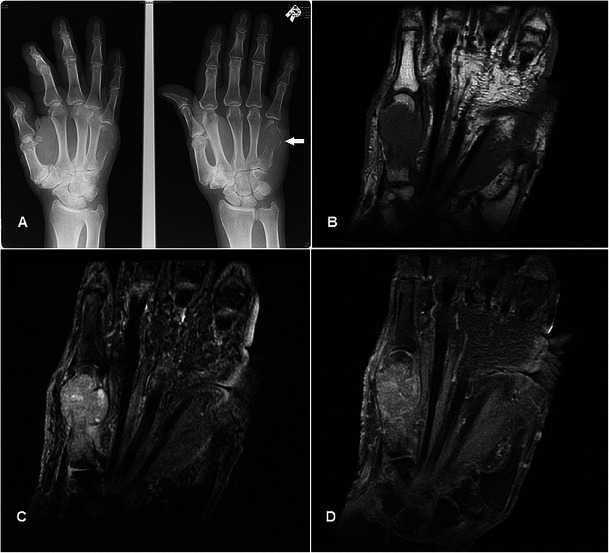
Bony metastasis in a 67-year-old male with a history of adenocarcinoma of the lung, presenting with a 2-week history of swelling and pain over the medial aspect of the right hand dorsum. (a) Plain radiograph shows permeative destruction of the fifth metacarpal (arrow). (b) T1w sequence shows destruction of the fifth metacarpal with infiltrative soft tissue, predominantly of heterogeneous intermediate signal. (c) The lesion is heterogeneously hyperintense on T2w-FS sequence. (d) Heterogeneous enhancement is seen extending to the adjacent soft tissues
